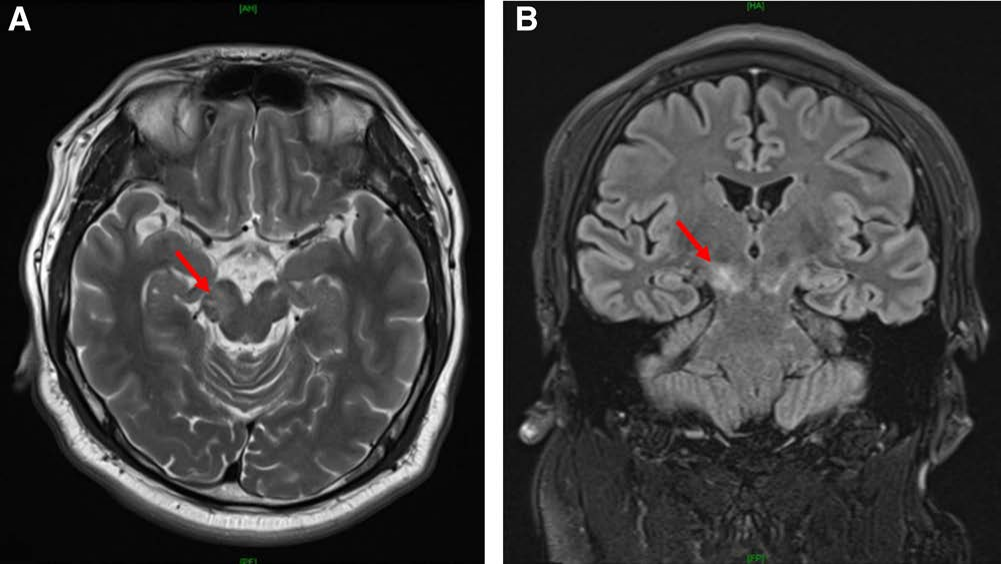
Brain magnetic resonance imaging of the right midbrain in T2 weighted image. (A) Axial image, (B) Coronary image. Red arrows showed ill-defined patchy hyperintense lesion in the right midbrain in T2 weighted image.
